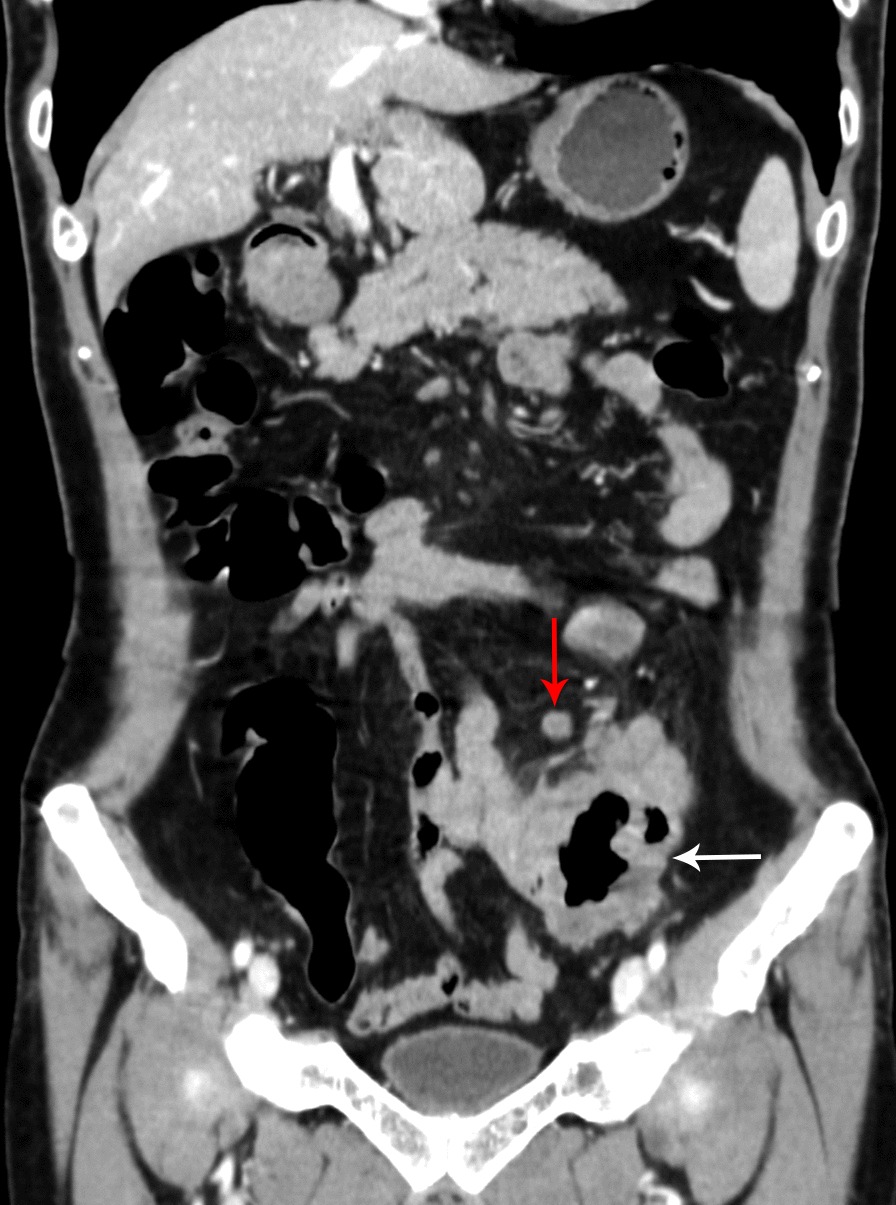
Abdominal CT. Abdominal CT shows a mass communicated with the small intestinal lumen (white arrow), and the outer and inner margins of the mass are irregular. A lymph node involvement is observed in the adjacent mesentery (red arrow)
